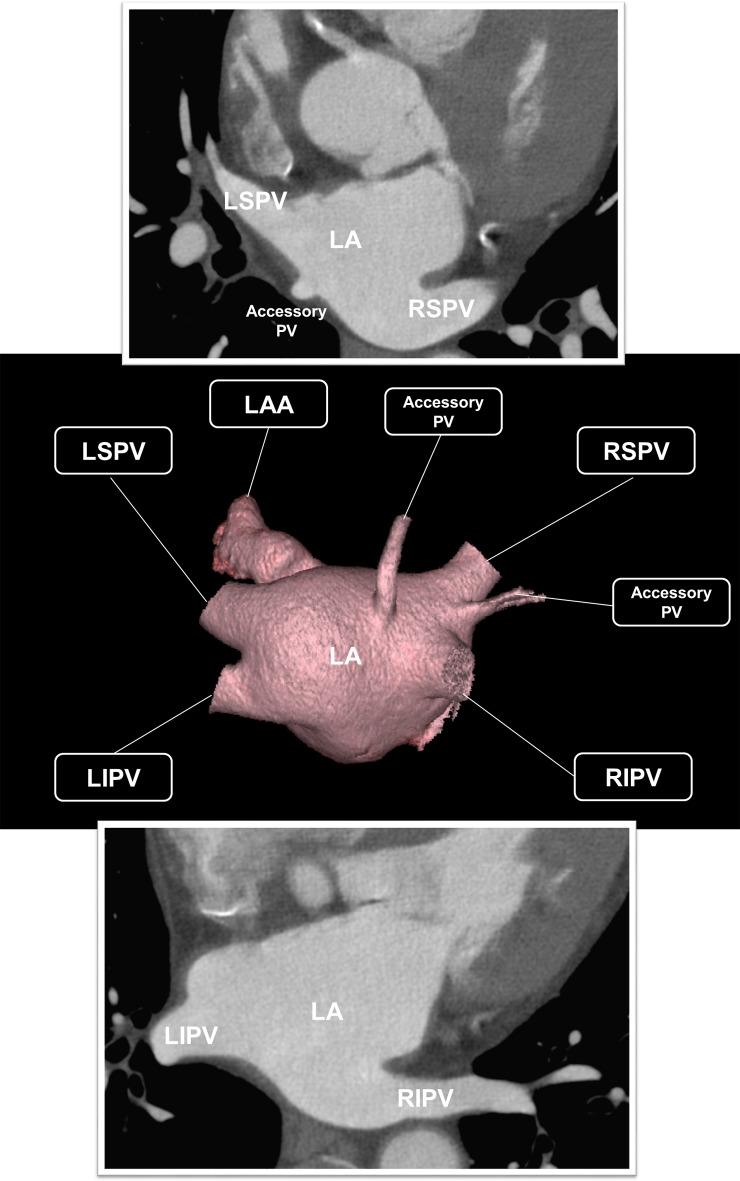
Anatomy of the LA and PVs at CCT using the axial images and 3D reconstruction. The most common configuration features four distinct PV ostia in the posterior LA on the right, the right superior and inferior PVs (RSPV and RIPV) have separate ostia, often divided by the LA wall, while on the left, the superior and inferior PVs (LSPV and LIPV) typically share closely positioned ostia without such separation. An accessory PV draining separately into the LA from the ipsilateral superior and inferior PV creates additional ostia. These accessory PV, often named based on the pulmonary segment they drain, are more commonly found on the right side than on the left, with an incidence reported in up to one-fourth of the population. When present, accessory PV trunks are typically shorter and narrower than the main PVs and may sometimes cross pulmonary fissures. LA; left atrium, PV; pulmonic vein, CCT; cardiac computed tomography, 3D; three-dimensional, LAA; left atrial appendage
